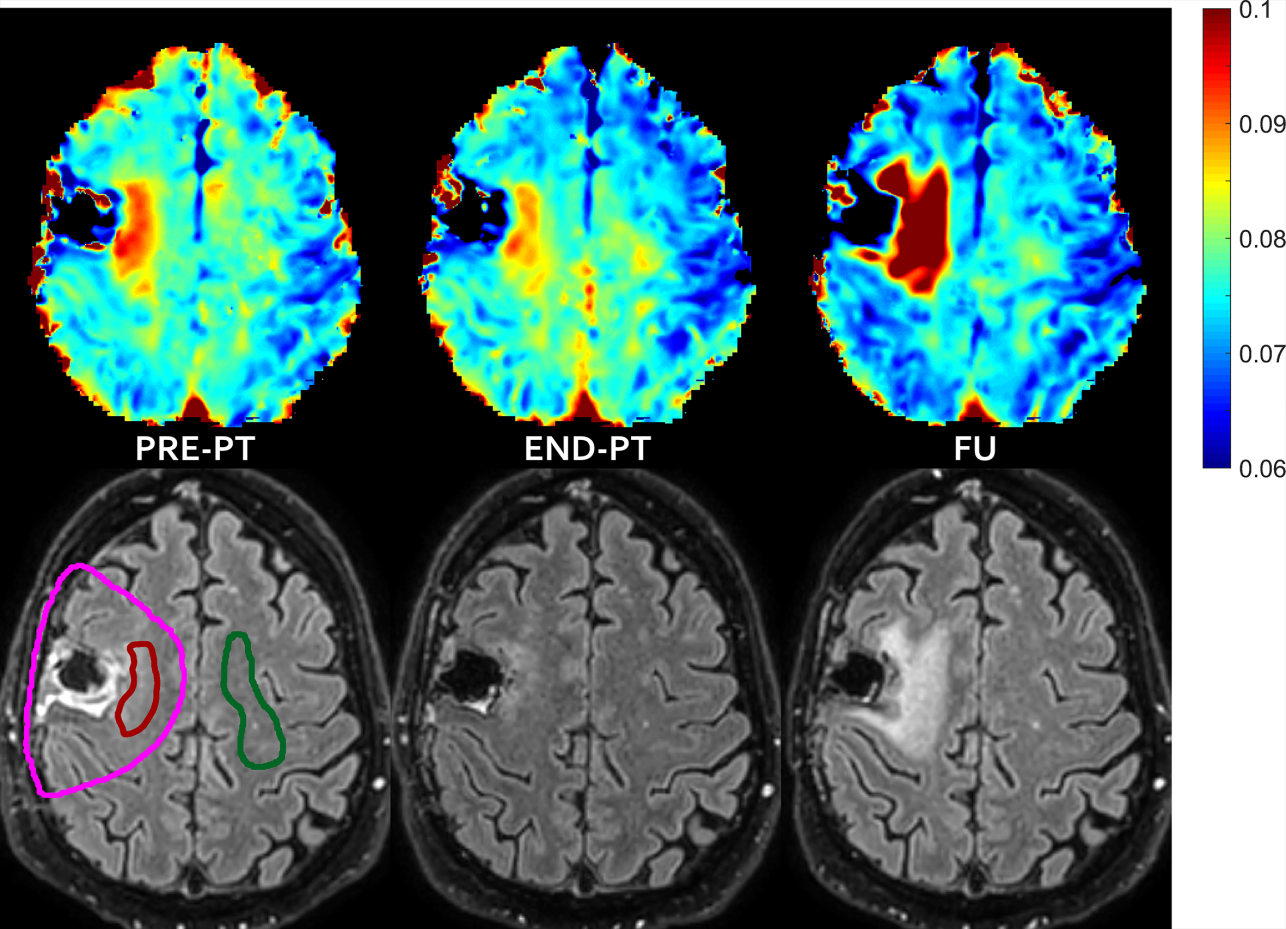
FLAIR increase pattern after temporary T2 decrease—In patient #2 the mismatch, i.e. the abnormal T2 area that extended outside hyper-intense FLAIR area before proton irradiation (left) evolved into new area of FLAIR hyper-intensity diagnosed as radiation induced damage at follow-up (right), with some partial temporary recovery at the end of the radiation treatment (middle). T2 intra/extracellular water maps (top) and FLAIR images (bottom) are shown. Colored bar is shown in seconds. On pre-PT FLAIR image the contours of the CTV (violet line), of the mismatch ROI (red line) and of the contralateral healthy ROI (green line) are shown.
